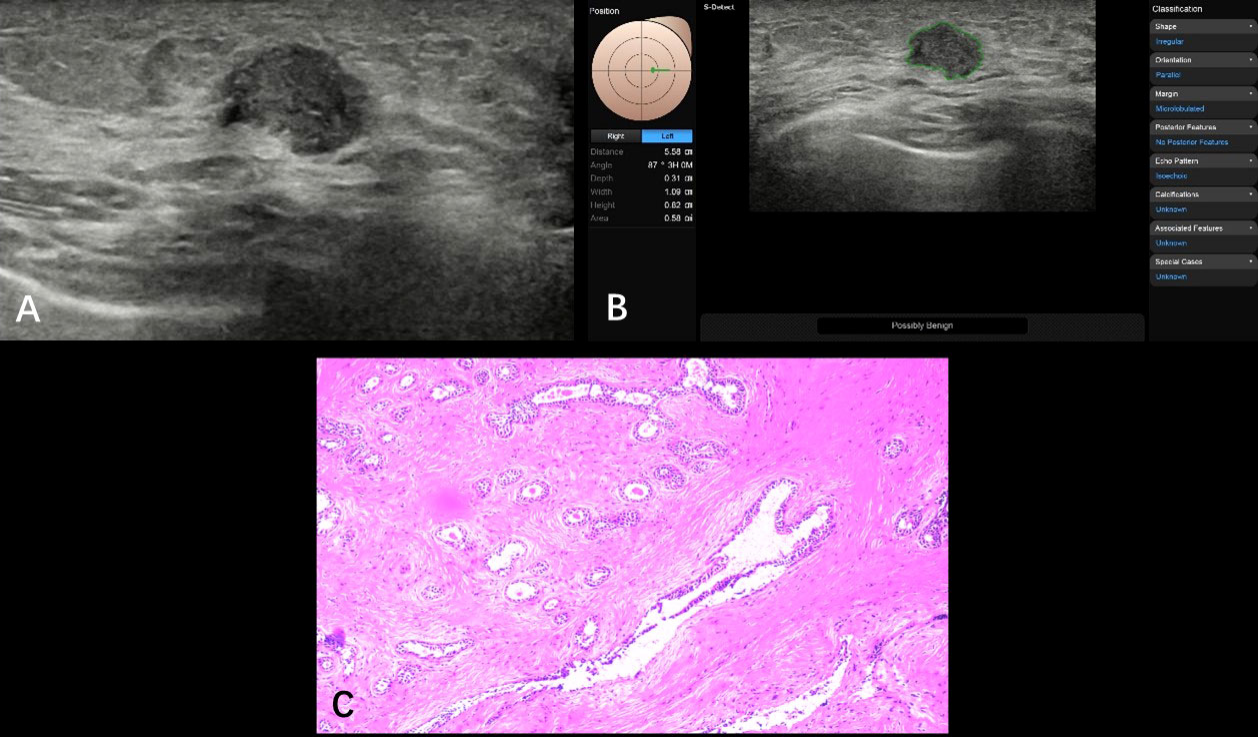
Fibroadenosis with fibroadenoma formation from a 35-year-old patient (BI-RADS 4a). A hypoechoic nodule (1.09 × 0.82 cm) was found at the 3 o’clock point of her left breast; ultrasound graphic showed glossy edge, irregular shape, partly angulated, and nonuniform internal echo (A). S-Detect artificial intelligence recommendation was “possibly malignant” and the BI-RADS score receded from 4a to 4b (B). Pathologically confirmed as breast fibroadenosis with fibroadenoma formation (C).
